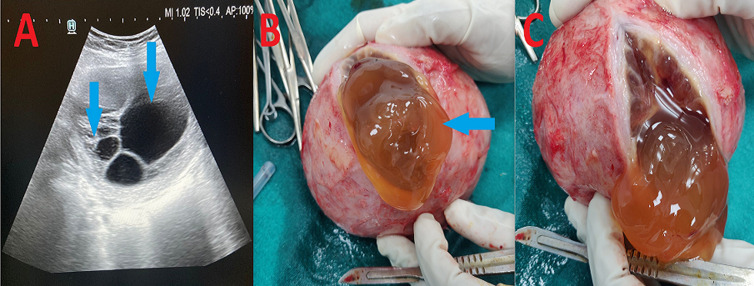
A) a complex cystic mass in the right adnexa with numerous internal septations on transabdominal and pelvis sonography; B, C) cystic degeneration of fibroid with straw-colored fluid oozing out
